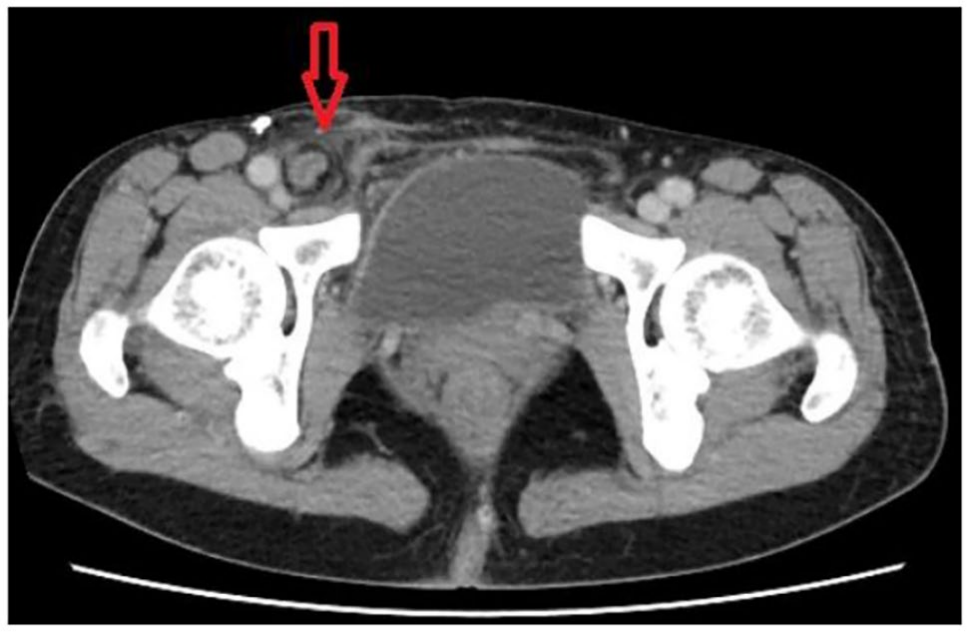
Axial plane of multidetector computed tomography image. A dilated appendix entrapped in femoral canal and some fluid collection
